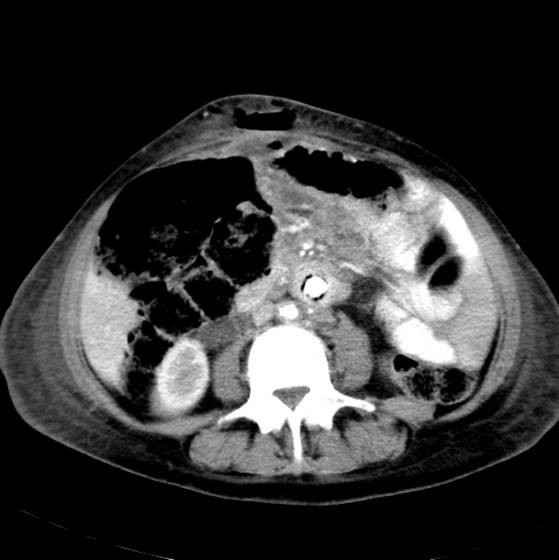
CT scan on 15^th ^postoperative day demonstrating a rim of air around the graft.
